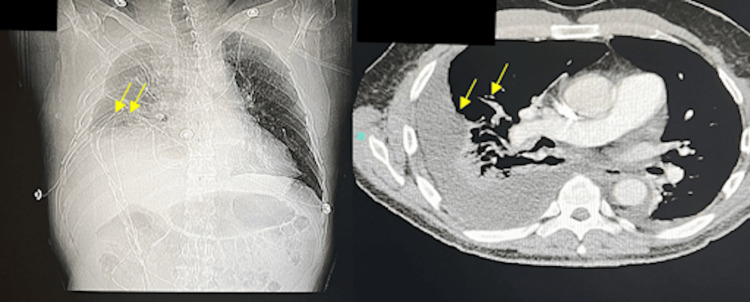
CT scan showing subsequent reaccumulation of pleural fluid following thoracentesis CT, computed tomography
